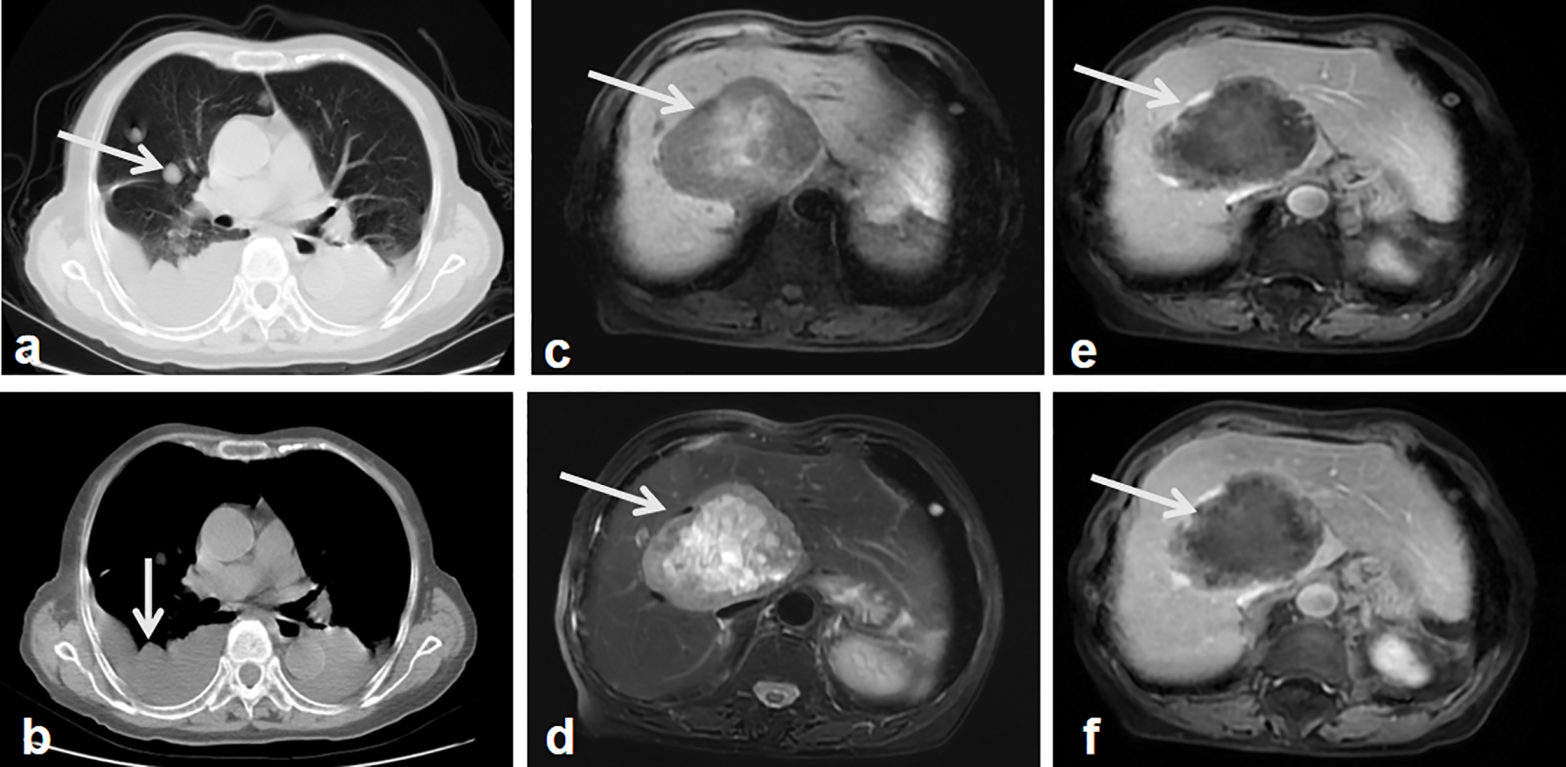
Follow-up images after surgery. Lung CT shows multiple nodules in the lung and thoracic effusion, which indicate the lung and possible pleural metastasis (A, B, arrow). MR T1 weighted images show a well-demarcated, heterogeneous hypointense signal tumor in the right lobe of the liver with patchy hypersignal intensities in the central of the tumor (C, arrow). MR weighted images show the tumor mass with heterogeneous hypersignal intensities (D, arrow). Contrast-enhanced MR weighted images of arterial (E), portal phase (F) show the tumor mass with intermediate enhancement of the peripheral component, the non-enhanced hypointense signal central area (arrows).
